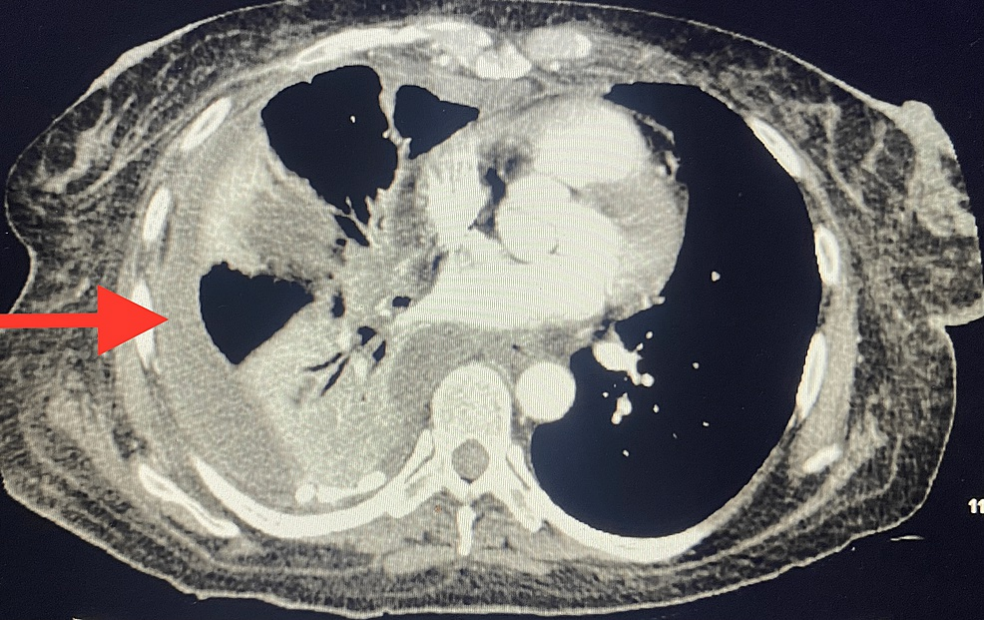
CT chest showing small loculated right-sided pleural effusion, interval development of left-sided pleural effusion, and linear opacities of atelectasis in the right middle lobe. The effusion is slightly smaller than those reported in prior studies. Stable right lower lobe consolidation demonstrates that the heterogeneous enhancement likely contained a small area of cavitation.
